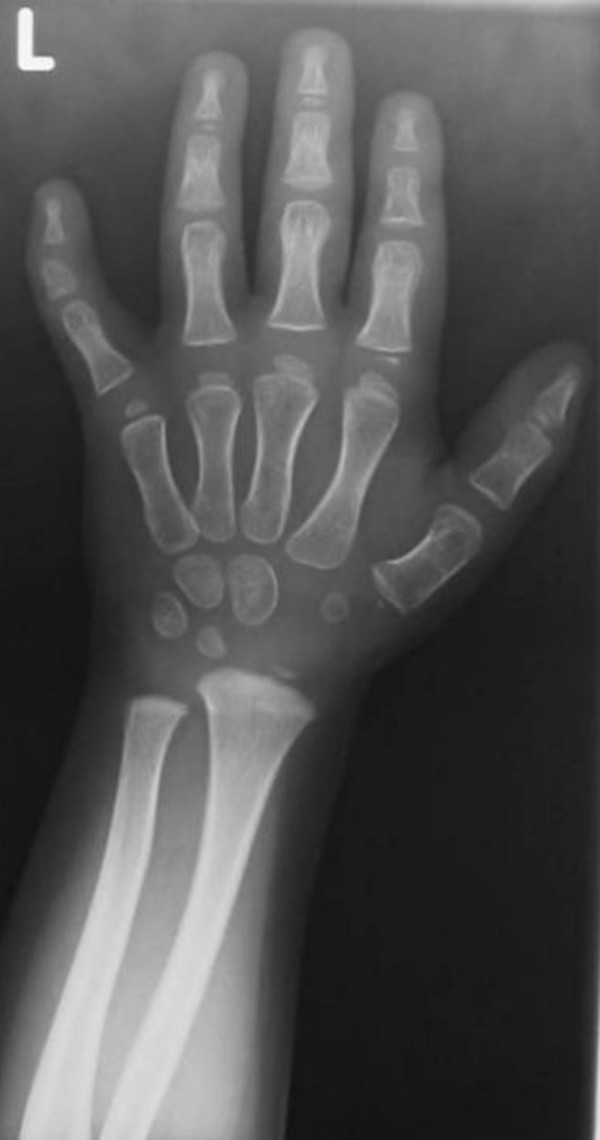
Anteroposterior hand radiograph at the age of 2- years showed advanced carpal ossification centres equivalent to bone age of 4 years 8 months, supernumerary ossicles at the proximal portion of the first metacarpal and another at the proximal portion of the middle phalanx of the second metacarpal. Delta like- middle phalanx of the fifth finger was evident.
